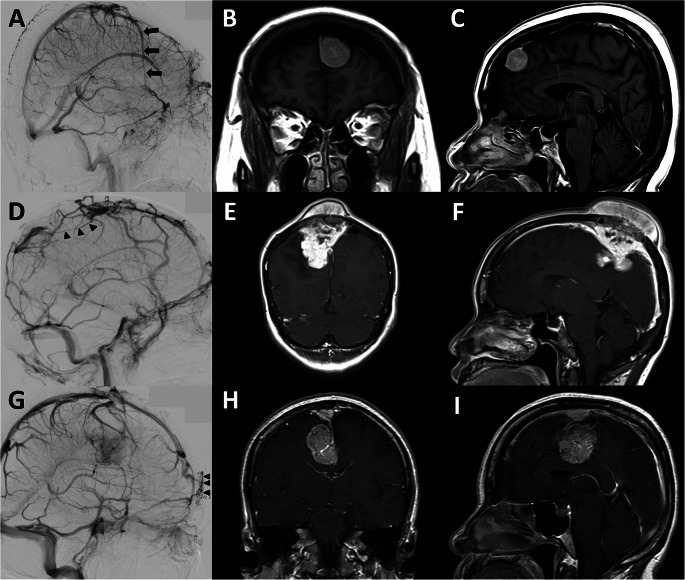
MV and other types of CVP. DSA and contrast-enhanced cranial MRI in case no.6 (A-C), 7 (D-F), and 8 (G-I). (A) MV (black arrows) accompanying the anterior branch of the MMA, running from the SSS to the sphenoparietal sinus on DSA; (B, C) contrast-enhanced cranial MRI in coronal and sagittal views, pathological diagnosis was solitary fibrous tumor; (D) cortical veins making a detour (black arrowheads) around the area of SSS obstruction on DSA; (E, F) contrast-enhanced cranial MRI in coronal and sagittal views, pathological diagnosis was metastatic adenocarcinoma; (G) sinus pericranii (black arrowheads) on DSA; and (H, I) contrast-enhanced cranial MRI in coronal and sagittal views, pathological diagnosis was meningioma. CVP, collateral venous pathway; DSA, digital subtraction angiography; MMA, middle meningeal artery; MV, meningeal vein; SSS, superior sagittal sinus
